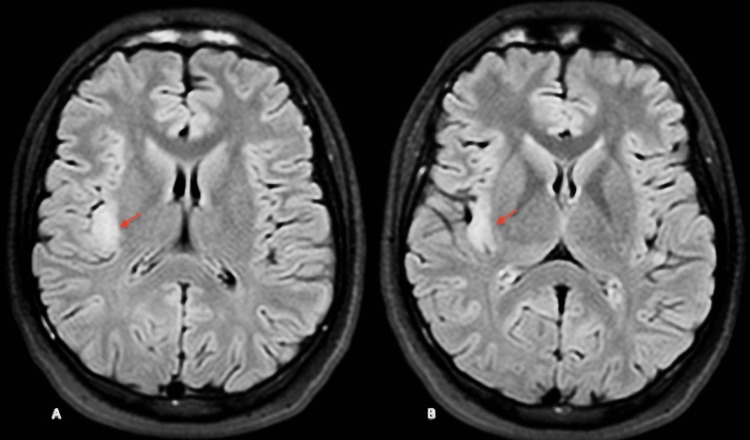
FLAIR sequence axial images showing right posterior insular focal cortical dysplasia FLAIR: fluid-attenuated inversion recovery
